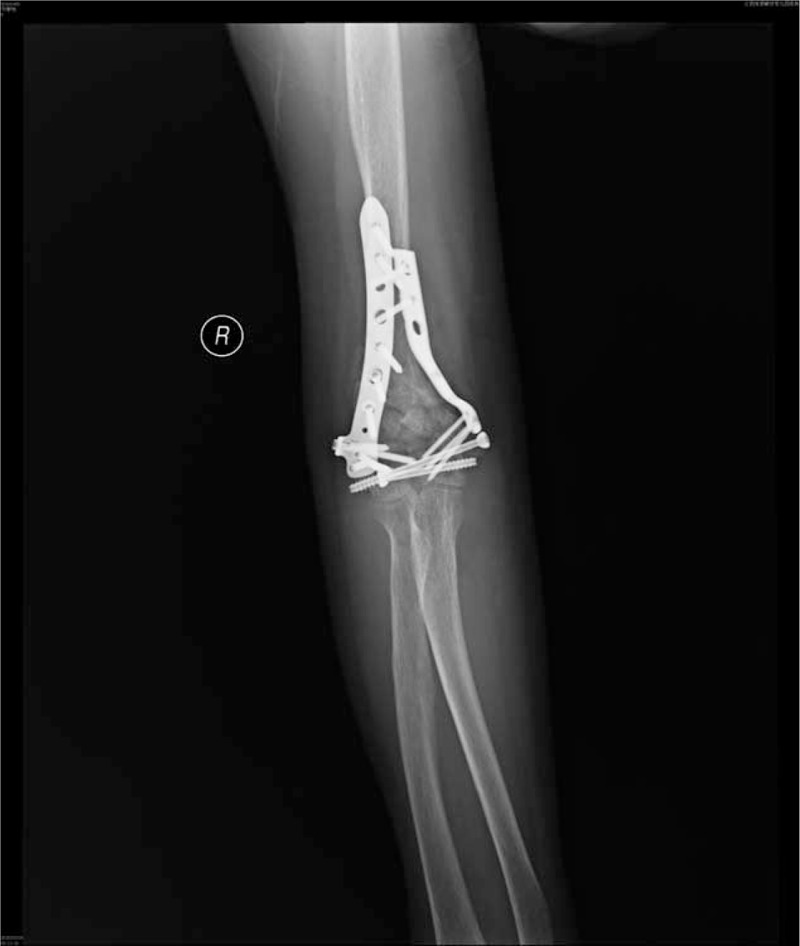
X-ray image showing callus formation at 6 weeks postoperative.
